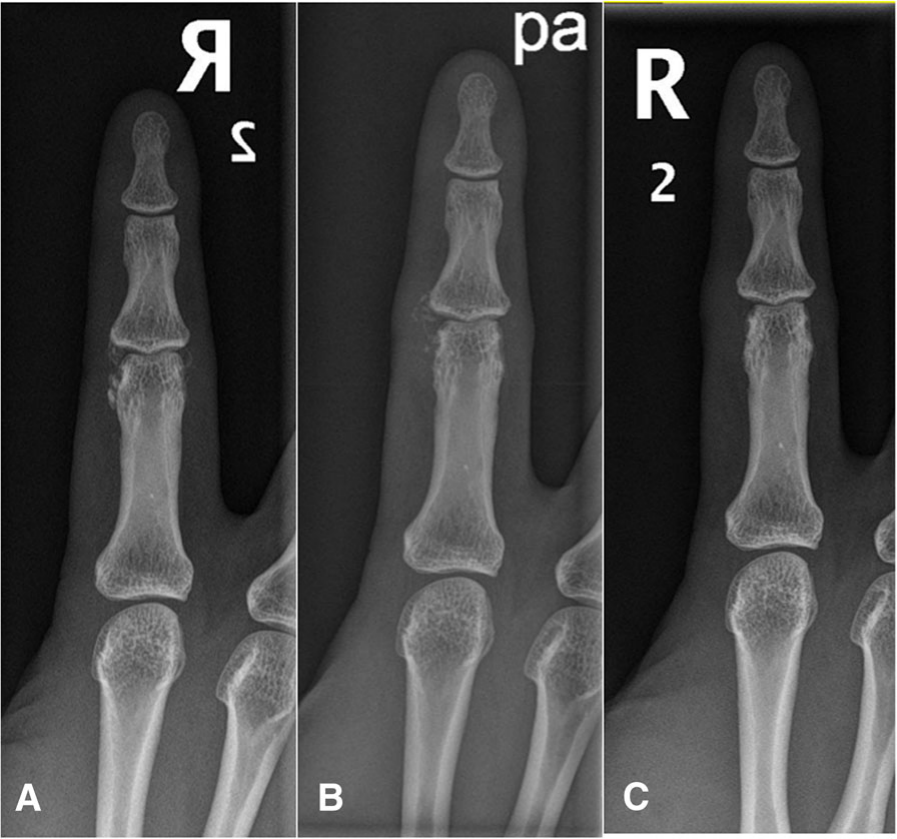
Patient (#9) with calcific periarthritis involving the PIP joints of the right second finger continued conservative treatment for 18 months and was pain-free at the last follow-up. X-rays taken at the initial visit (a) and at the 9- (b) and 18-month (c) follow-ups showed a marked reduction in calcification
